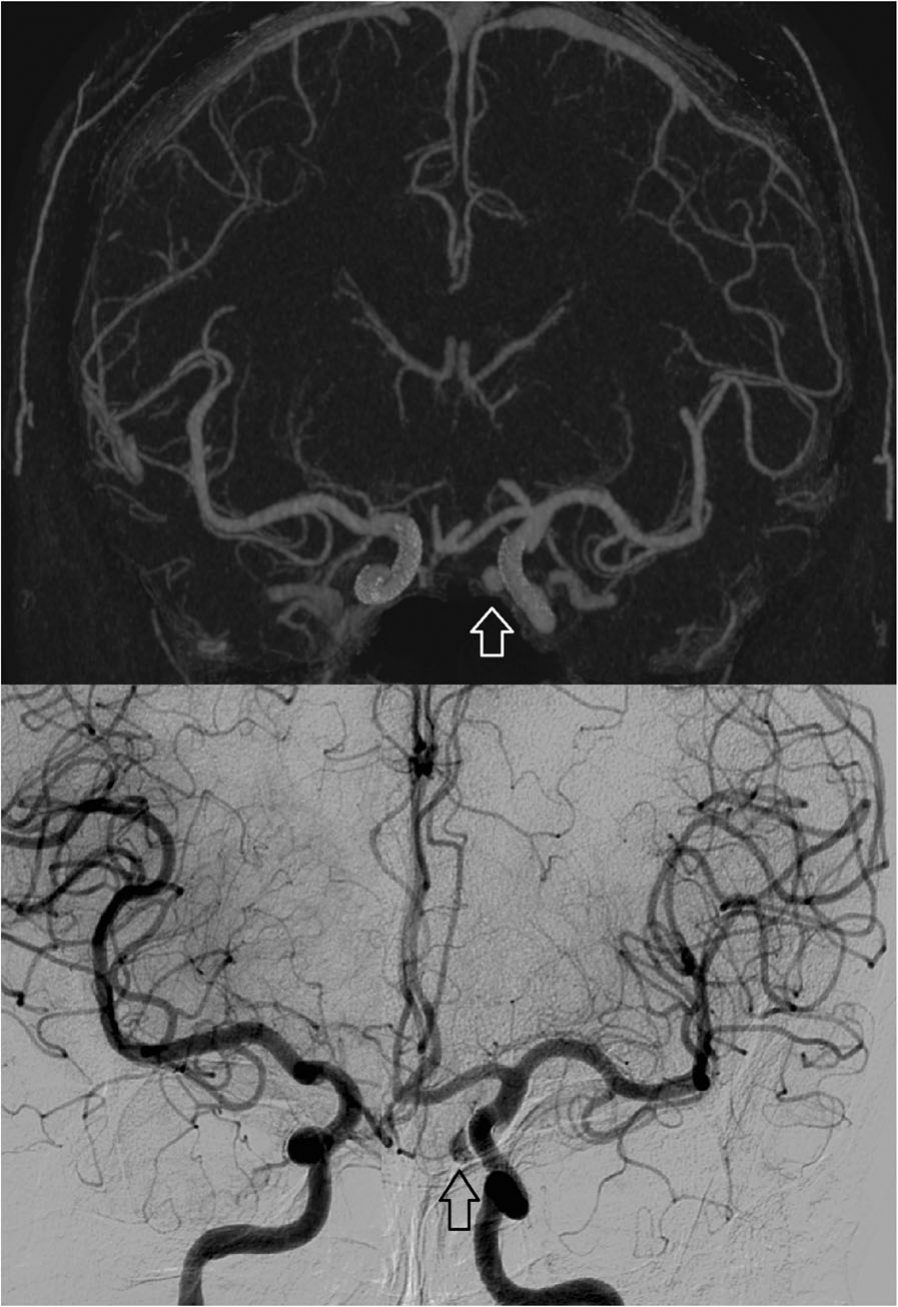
Fifty-seven-year-old female with flow diverter placement for treatment of internal carotid artery (ICA) aneurysms on both sides. At follow-up, occlusion of the right ICA aneurysm and residual contrast filling of the left ICA aneurysm (arrows) was seen with full consistency between UHR subtraction CTA (top) and conventional angiography (bottom)
